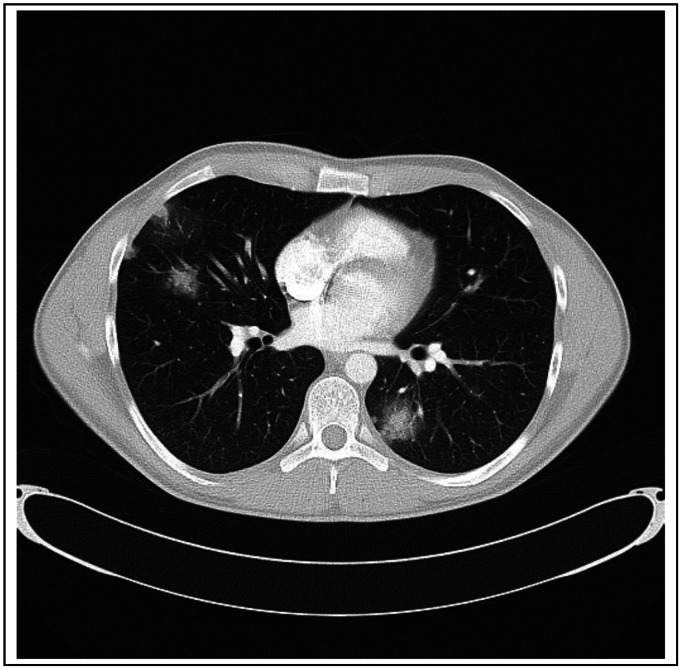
Multiple peripheral bilateral pseudonodular lesions with surrounding ground-glass-opacity halo in CT thorax scan.
